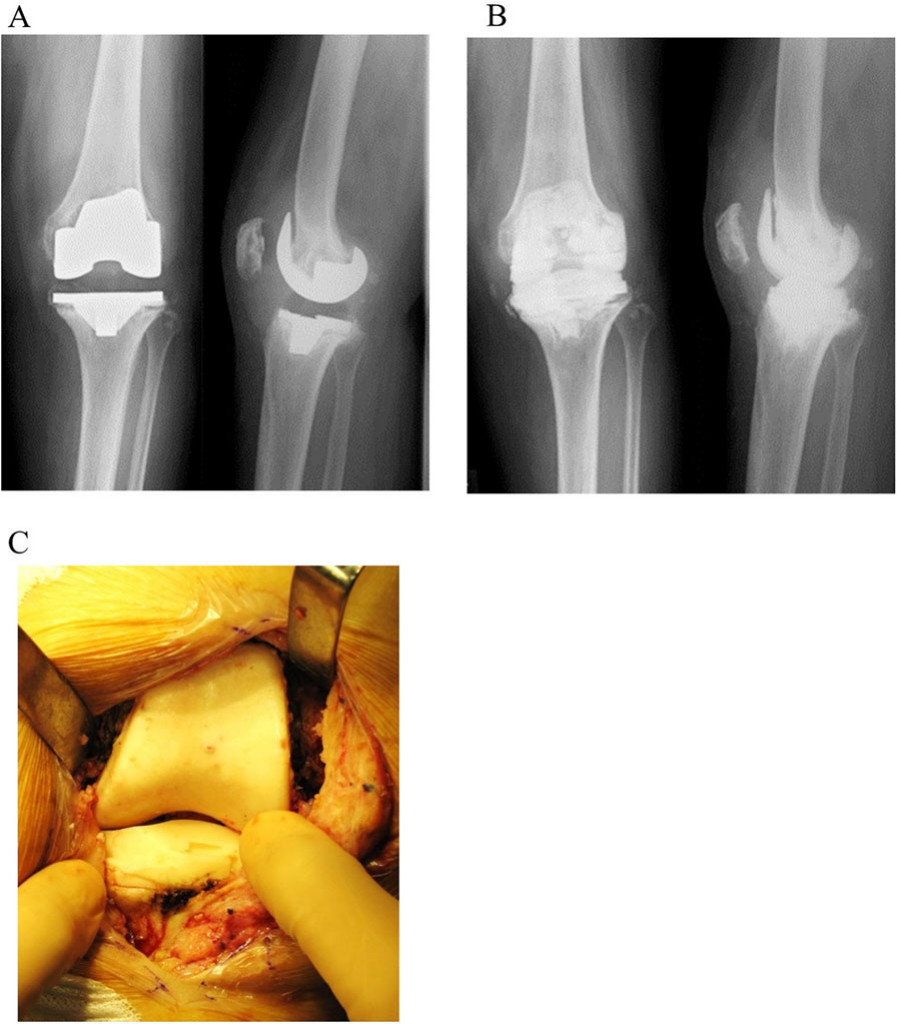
First-stage surgery: replacing antibiotic-loaded articulating cement spacers for periprosthetic joint infection. A Radiograph before the first surgery. Implant loosening was clearly observed. B Radiograph before the second surgery. Antibiotic-loaded articulating cement spacers were implanted. C Macroscopic image of articulating cement spacers
